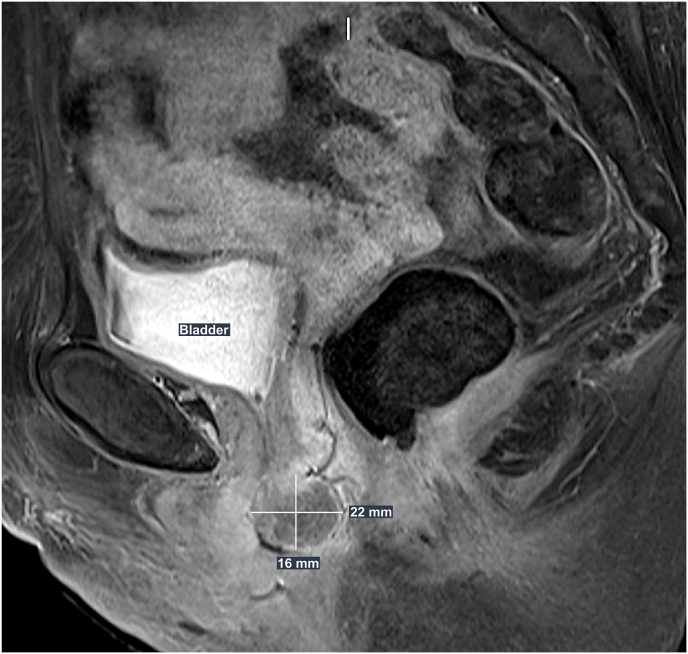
T1-weighted MRI pelvis. Sagittal view. A mass measuring 22mm × 16mm is identified in an intimate relationship to the inferior posterior urethra and the anterior vaginal wall. No enlarged pelvic nodes or groin nodules. This mass is concerning for malignancy.
